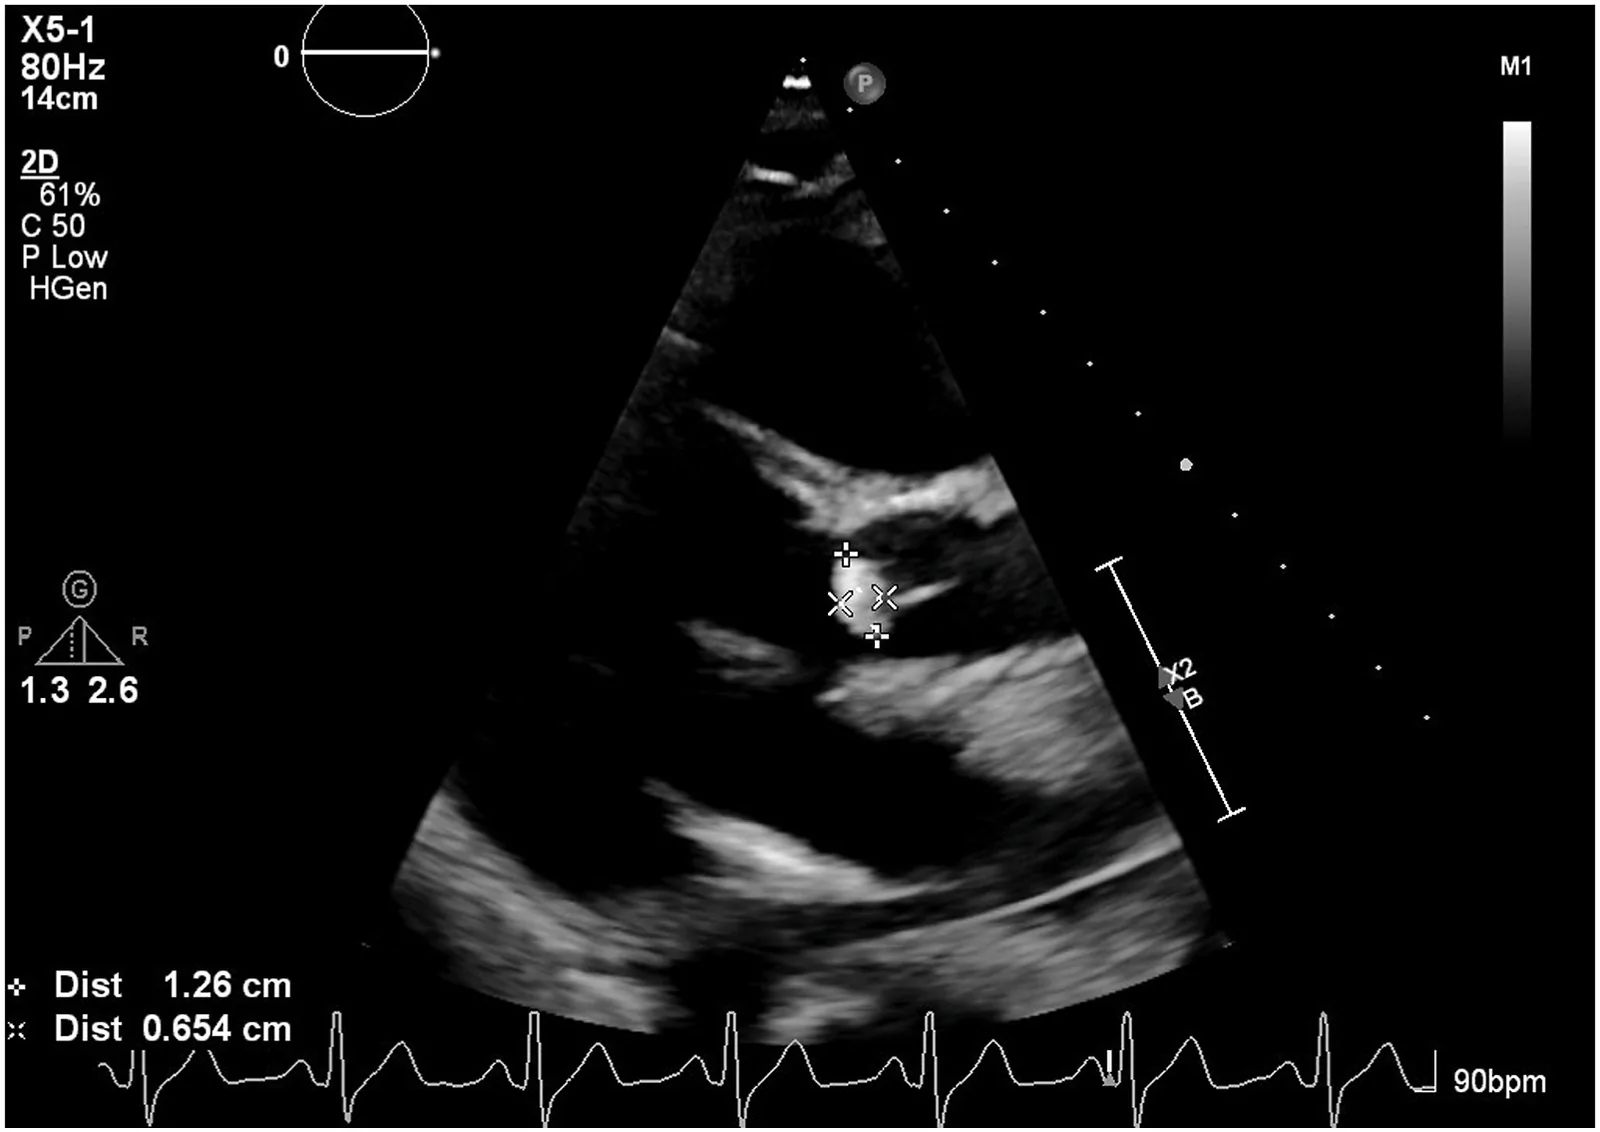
Two-dimensional TTE, parasternal long-axis diastolic view, demonstrates an irregular, sessile, echogenic mass (12.6 × 6.5 mm) attached to the ventricular surface of the noncoronary AV leaflet, consistent with a vegetation.
